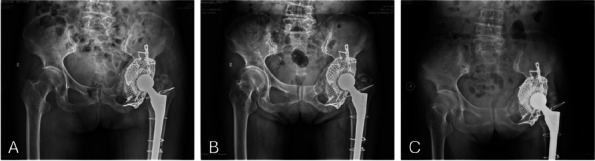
A, B and C Pictures showed postoperation, 3-month, and 6-month follow-up pelvic X-ray of the same case in Fig. 1
